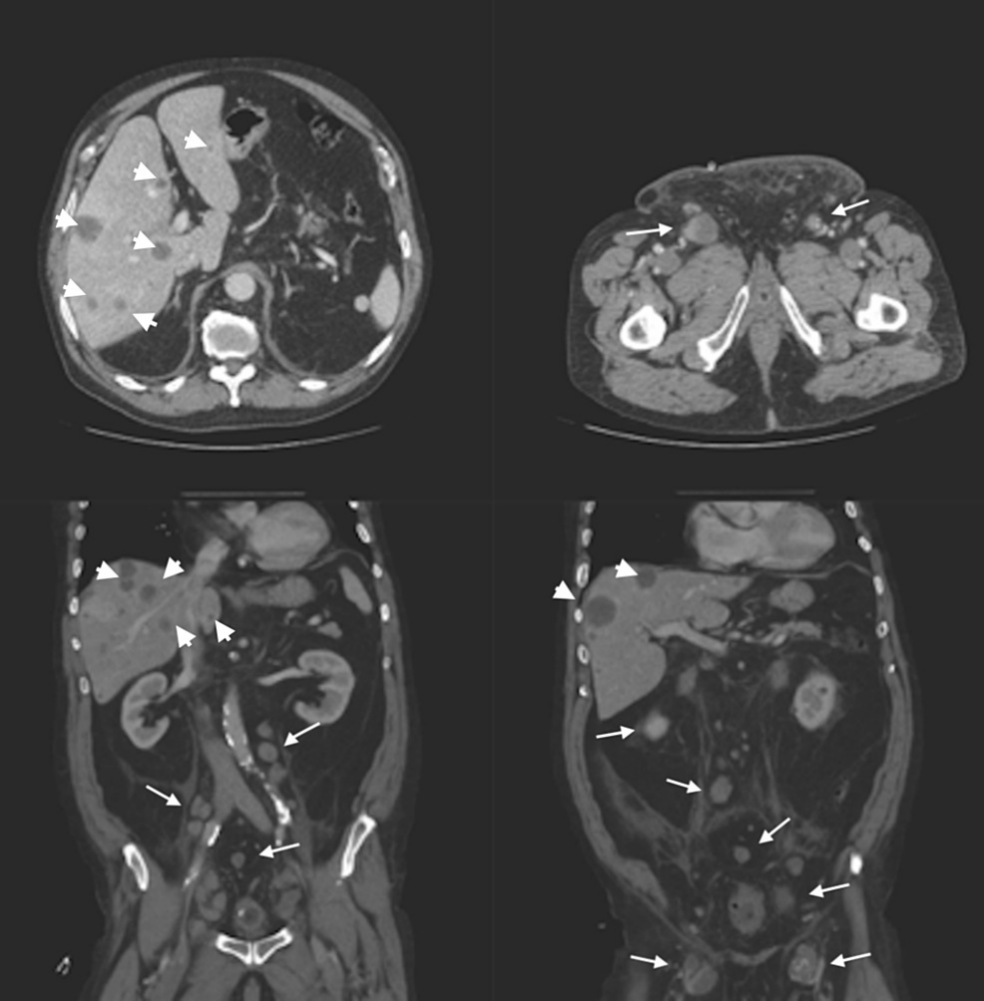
CT images The images show several hypodense nodules in both liver lobes suggestive of secondary lesions (thick arrows), and the presence of several retroperitoneal and groin pathological lymph nodes (thin arrows) CT: computed tomography
